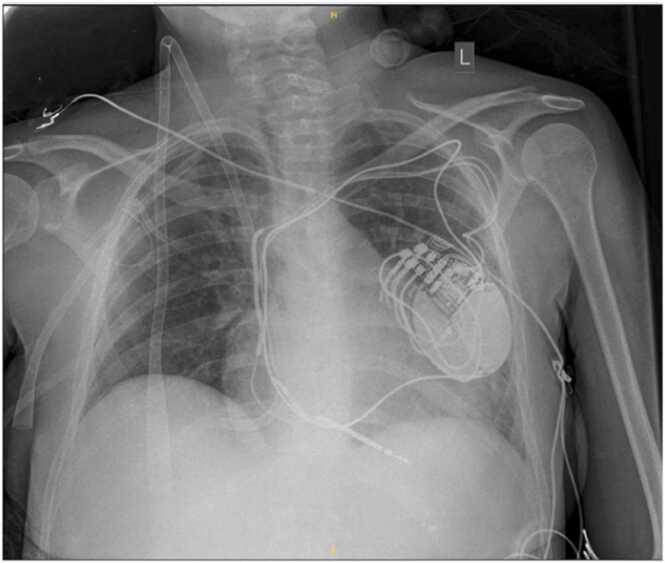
X-ray of patient supported by the “ECMO-TO-GO” concept.
